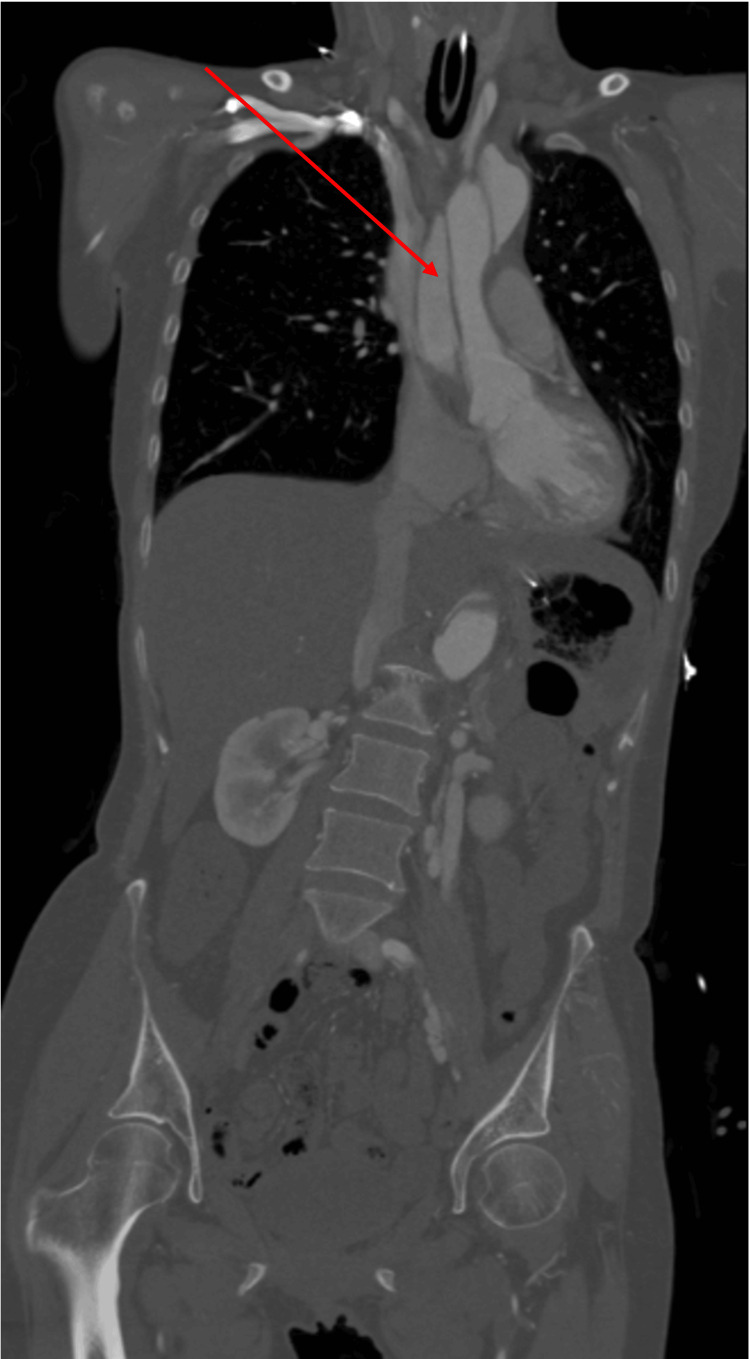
Coronal CT angiogram showing extensive propagation of the dissection from the aortic root through the thoracoabdominal aorta with involvement of major branch vessels.
